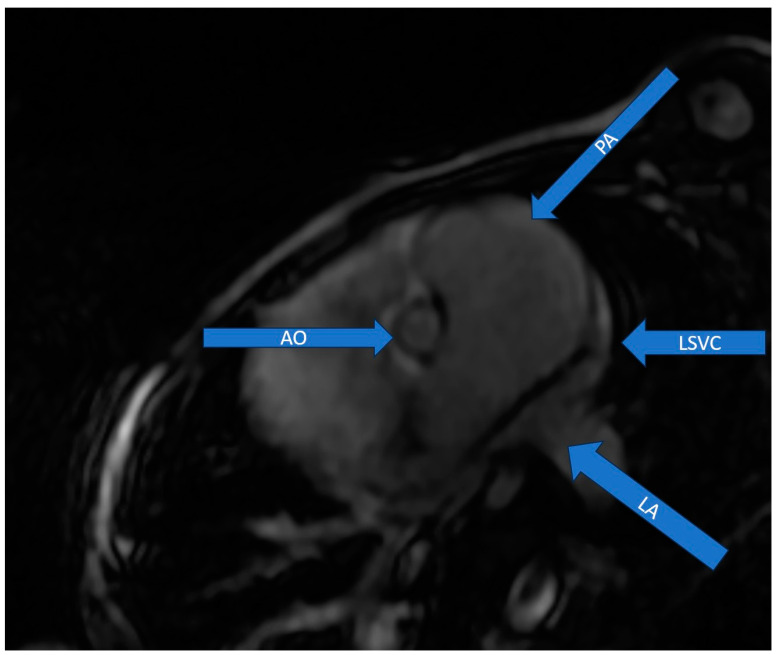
MRI: LSVC drains into the left atrium. Legend: AO—aorta; LA—left atrium; LSVC—left superior vena cava; PA—pulmonary artery.
